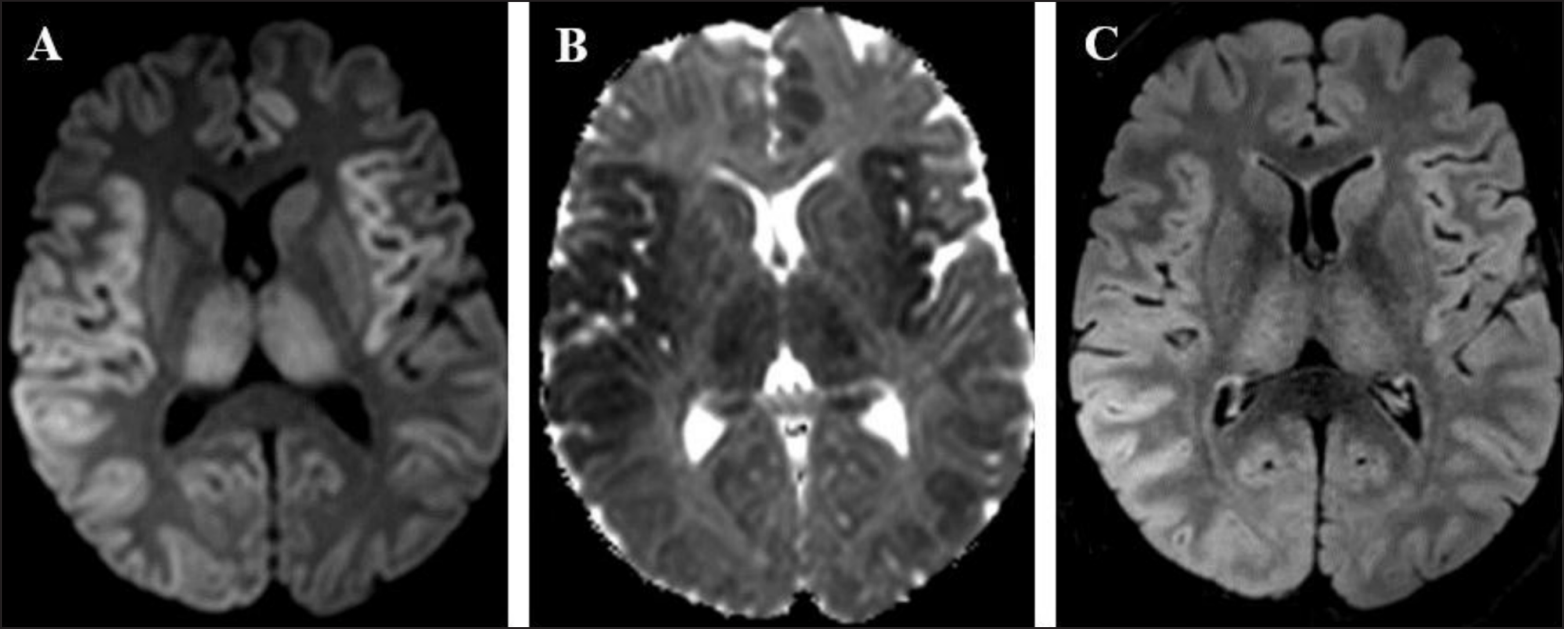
A 45-year-old man presented with seizures and fulminant hepatitis with hepatic failure, secondary to acetaminophen intoxication. (A) DWI (b-value = 1000 s/mm^2^) and (B) ADC map show cortical signal abnormalities in bilateral insulas and parietal lobes, left cingulate gyrus and both thalami, respectively high and low signal, consistent with cytotoxic edema. (C) FLAIR sequence shows high signal in the right parietal cortex and bilateral thalami.
